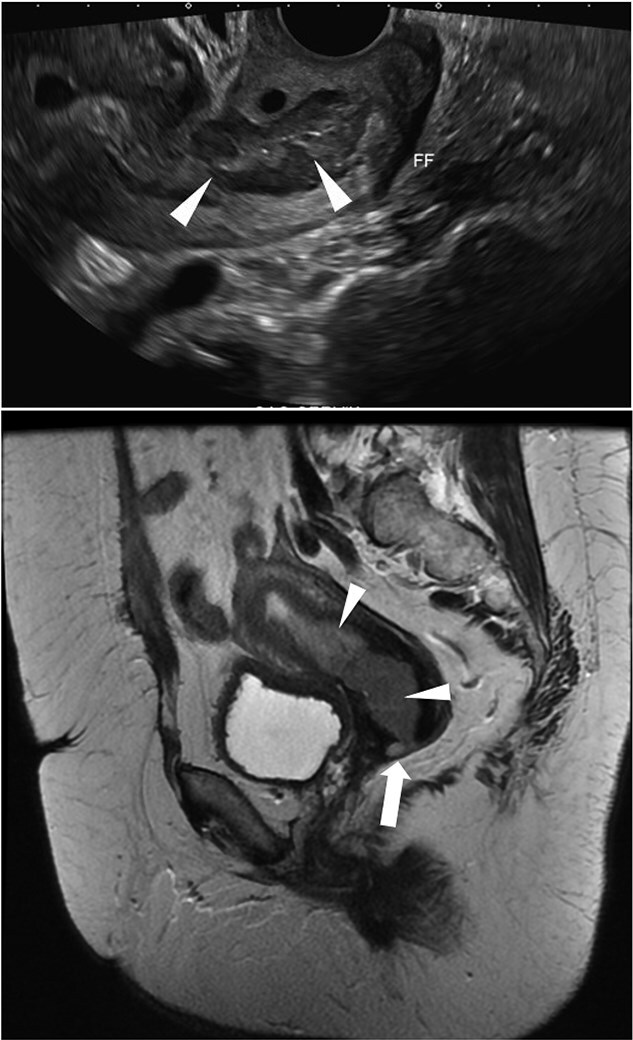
MRI: Pelvic MRI demonstrates a lobular soft tissue mass within the endometrial canal (arrowheads). The lesion extends from the superior endometrium to the level of the external os of the cervix with likely cervical stromal invasion (arrow).
